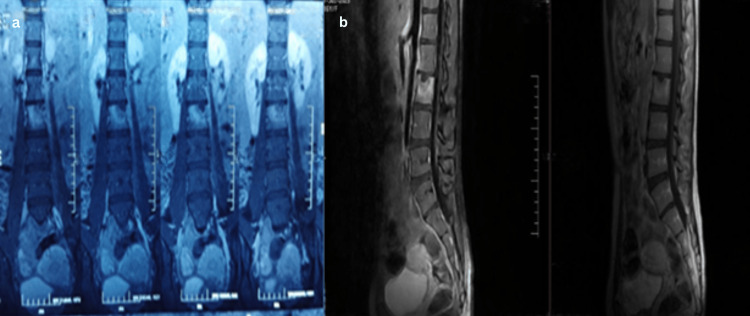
Spinal case 6 (a) Plain MRI of the lumbosacral region in the coronal view showing marrow intensity changes in both L1 and L2, extending to the cortical defect of the L2 superior endplate. (b) Contrast MRI in the sagittal view showing subtle post contrast enhancement in the anterior aspect of L1 inferior endplate and peripherally enhancing. Left paravertebral and prevertebral collection extending to the cortical defect of the L2 superior endplate. Data shown are representative MRI findings from a single patient case (spinal case 6). Original images obtained by the authors at Subbaiah Institute of Medical Sciences, Shivamogga.
